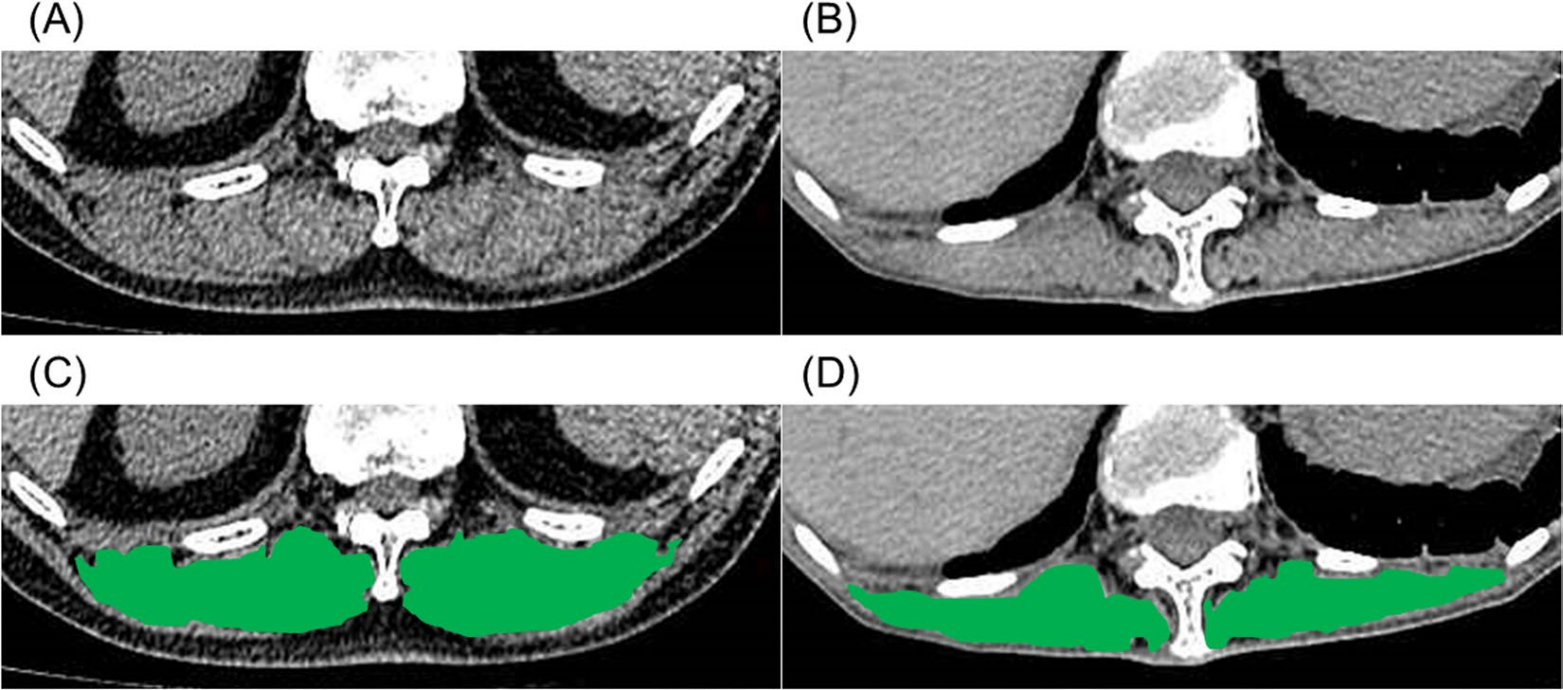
The cross-sectional area of the erector spinae muscles. Representative computed tomographic images used to measure the cross-sectional area of the erector spinae muscles (A,B). The cross-sectional areas of the erector spinae muscles are in green (C,D). The sums of the areas of the erector spinae muscles were 55.8 cm^2^ (A,C), and 14.9 cm^2^ (B,D).
